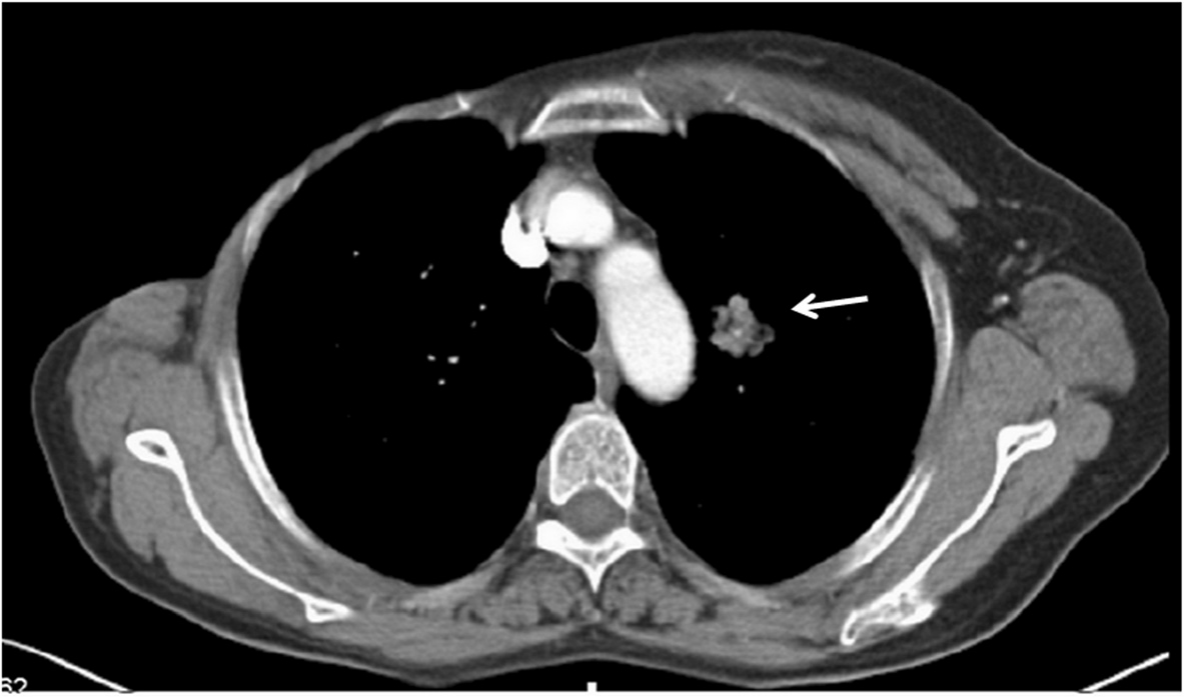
Enhancement computed tomography scan of chest reveals a 2.3 × 2.3 cm lobulated tumor in the left lung.
